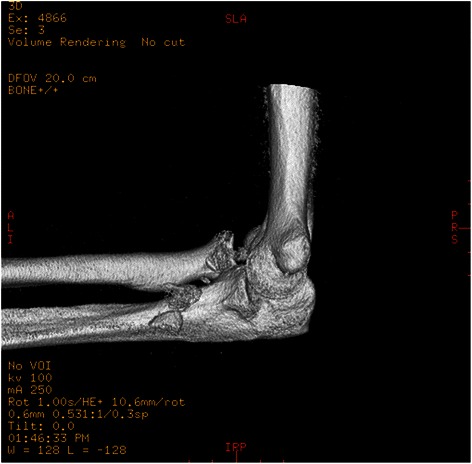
Computed tomography (three-dimensional reconstruction)
